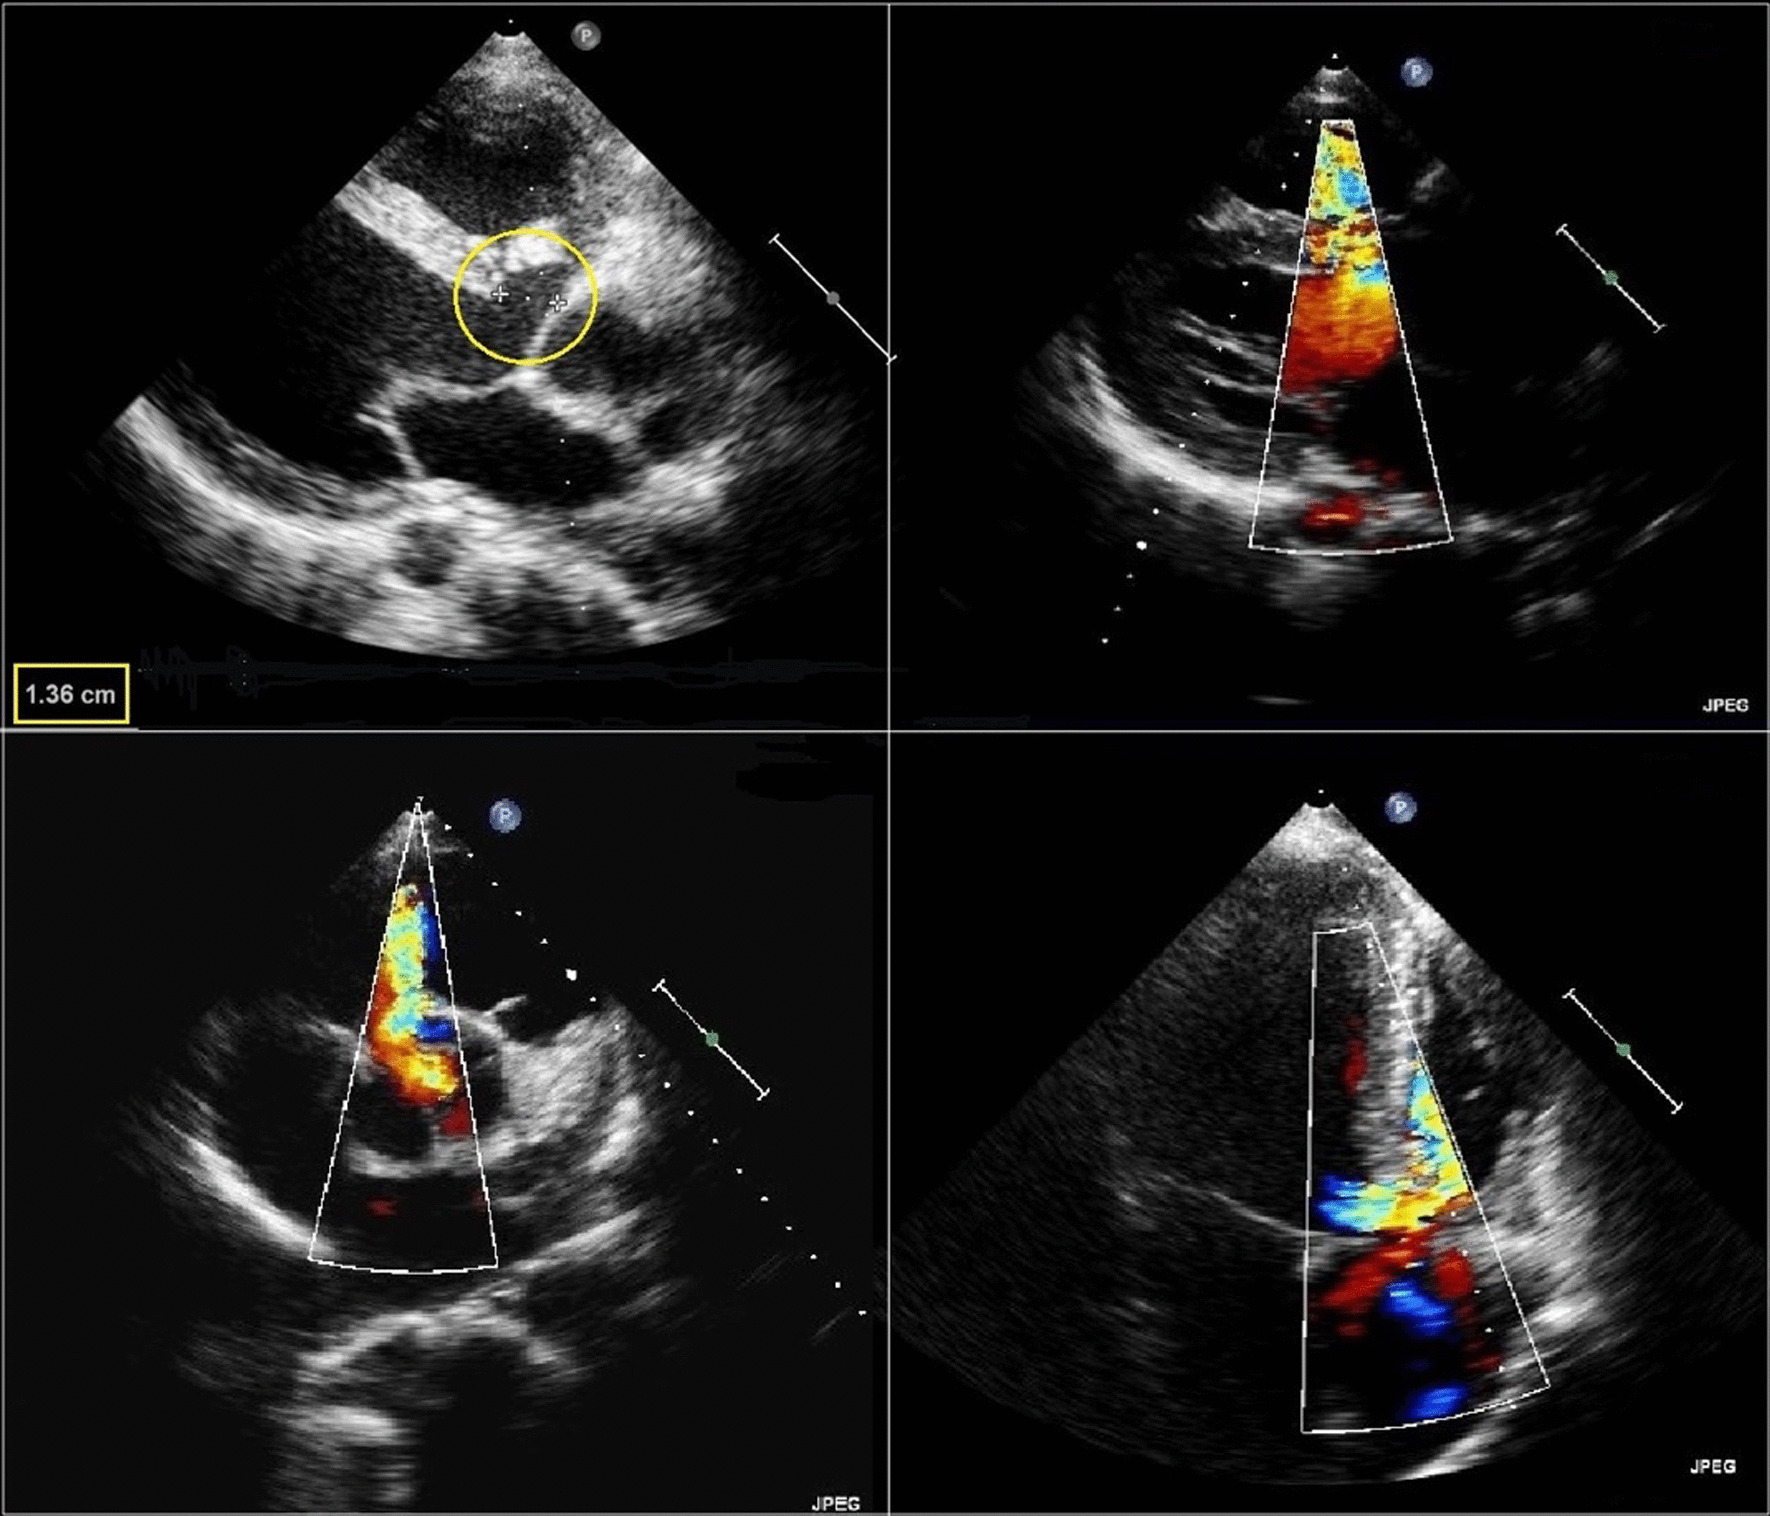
Color-Doppler imaging of ventricular septal defect in the parasternal and apical wiew
